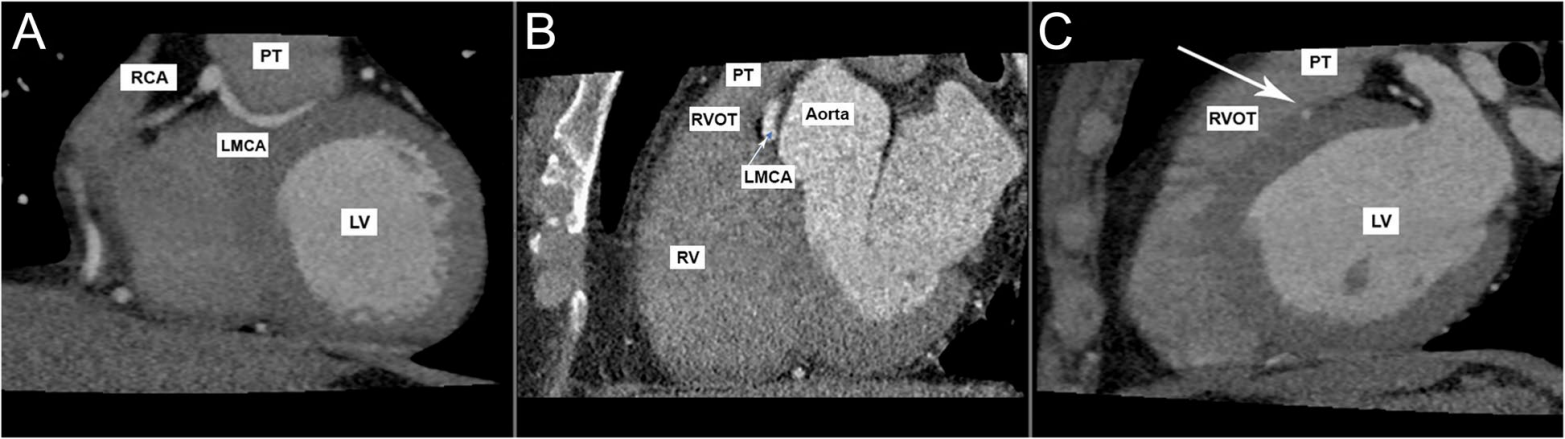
Computed Tomography Coronal and Sagittal View. a CT Coronal view demonstrating the common origin of RCA and LMCA with the sub-pulmonic course of LMCA below pulmonary trunk (PT). b CT reformatted sagittal view showing LMCA ‘malignant’ course in between PT and Aorta. c Computed Tomography Sagittal view showing the deep septal course of LMCA (arrow) within LV wall
